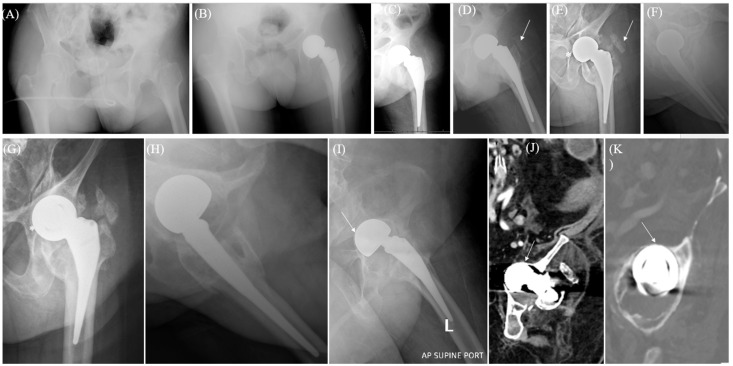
(A) Plain hip radiographs of a 47.1-year-old man with spastic cerebral palsy and limited indoor activities (Case 9, Table 4) who presented with a displaced left femoral neck fracture. (B) Immediate postoperative plain hip radiographs after cementless bipolar hemiarthroplasty. (C,D) Six-week postoperative plain pelvic radiographs showing stable components and early heterotopic ossification (HO) (arrow). (E,F) Here, 24-month postoperative plain hip radiographs showing HO (arrow) and prosthetic head protrusion through the ilioischial line (asterisk*). (G,H) A 62-month plain hip radiograph showing grade 3 HO and prosthetic head protrusion (asterisk*). At this point, the patient presented with marked groin pain (I–K) 177-month postoperative radiographs (I) and CT scan cuts (coronal (J) and sagittal (K)) showing an almost completely fused hip with prosthetic head intrapelvic protrusion (arrows) and class 4 HO.
